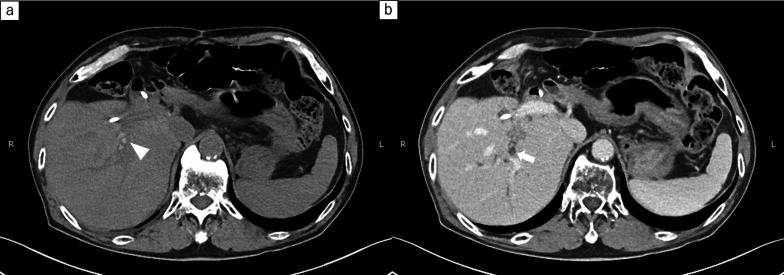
Computed tomography (CT) images of Case 1. a Plain CT, b Enhanced CT revealed intrahepatic lithiasis (arrowhead) and intrahepatic biliary dilation (arrow)
